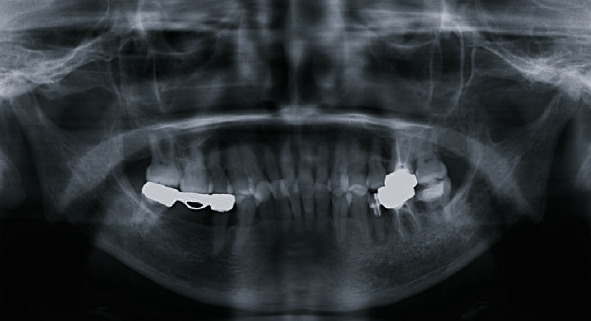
Panoramic radiograph approximately 2 years after the surgery. There are no findings suggesting recurrence.
